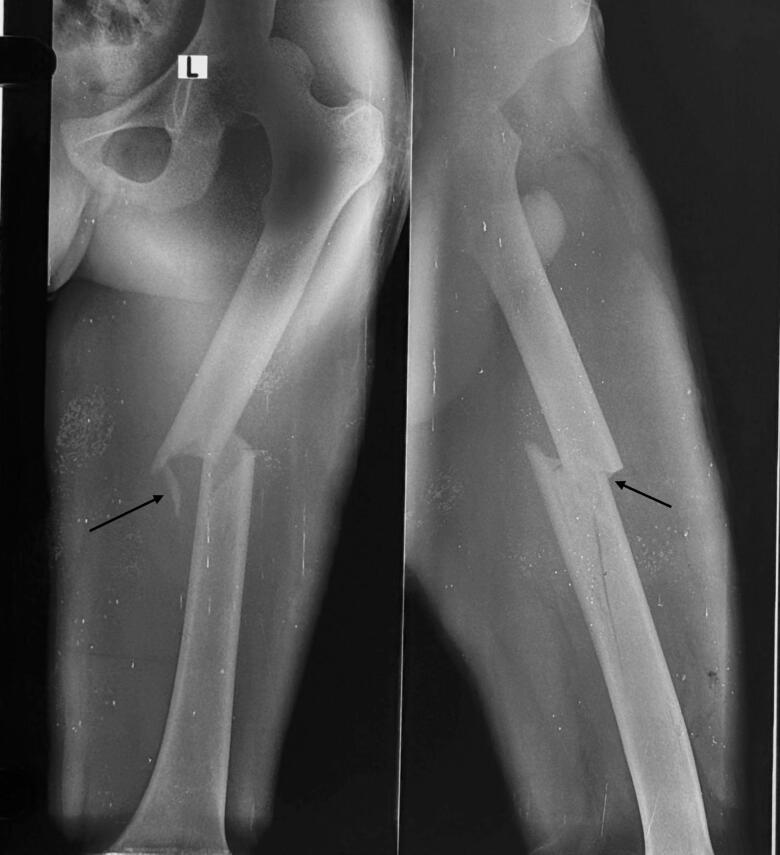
Pre-operative anteroposterior and lateral x-ray of femur showing mid-shaft femur fracture with a small fragment.
